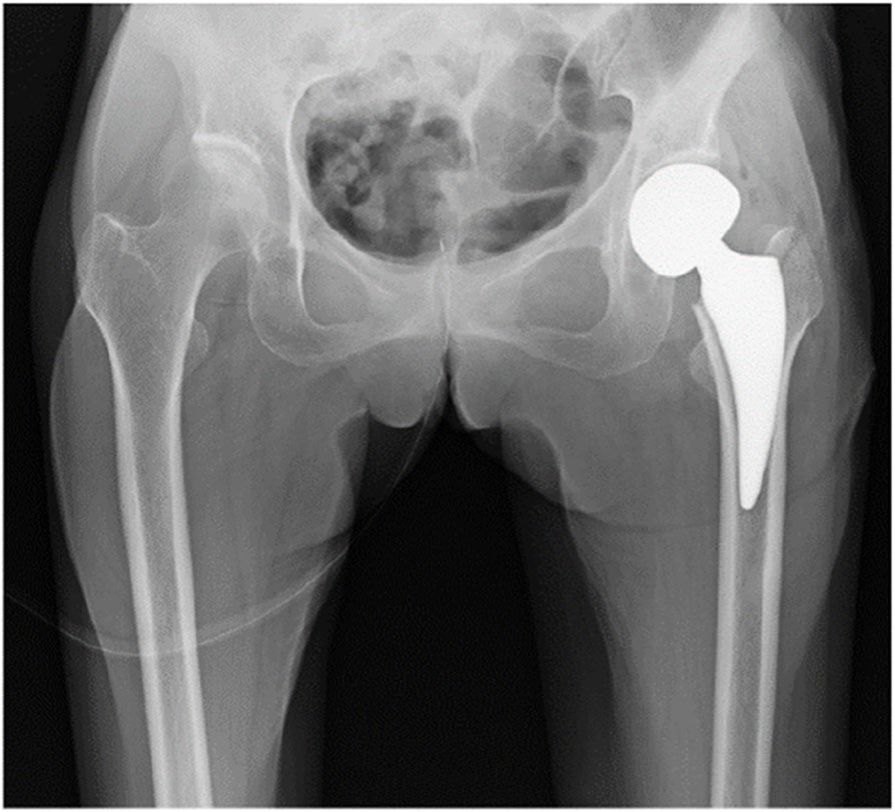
Postoperative hip plain X-rays of a representative case. Case. 81-year-old female diagnosed with a femoral neck fracture. Ambulatory status before the injury was Level 1
